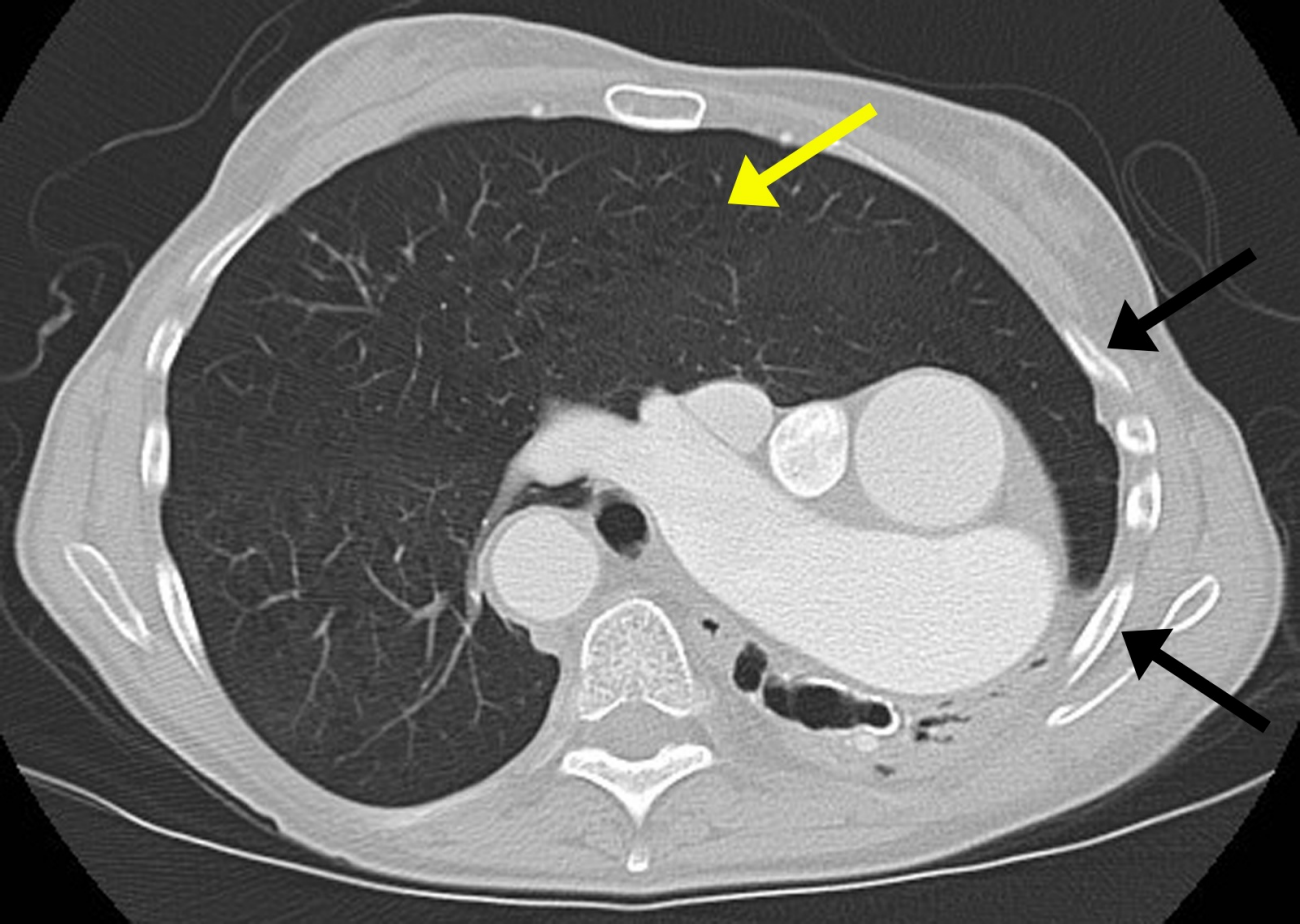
Contrast-enhanced CT chest, axial image in lung window. There is hyperinflation and expansion of the right lung, almost occupying the entire thoracic cavity (yellow arrow). The left chest is asymmetrically smaller when compared to the right (black arrow).
